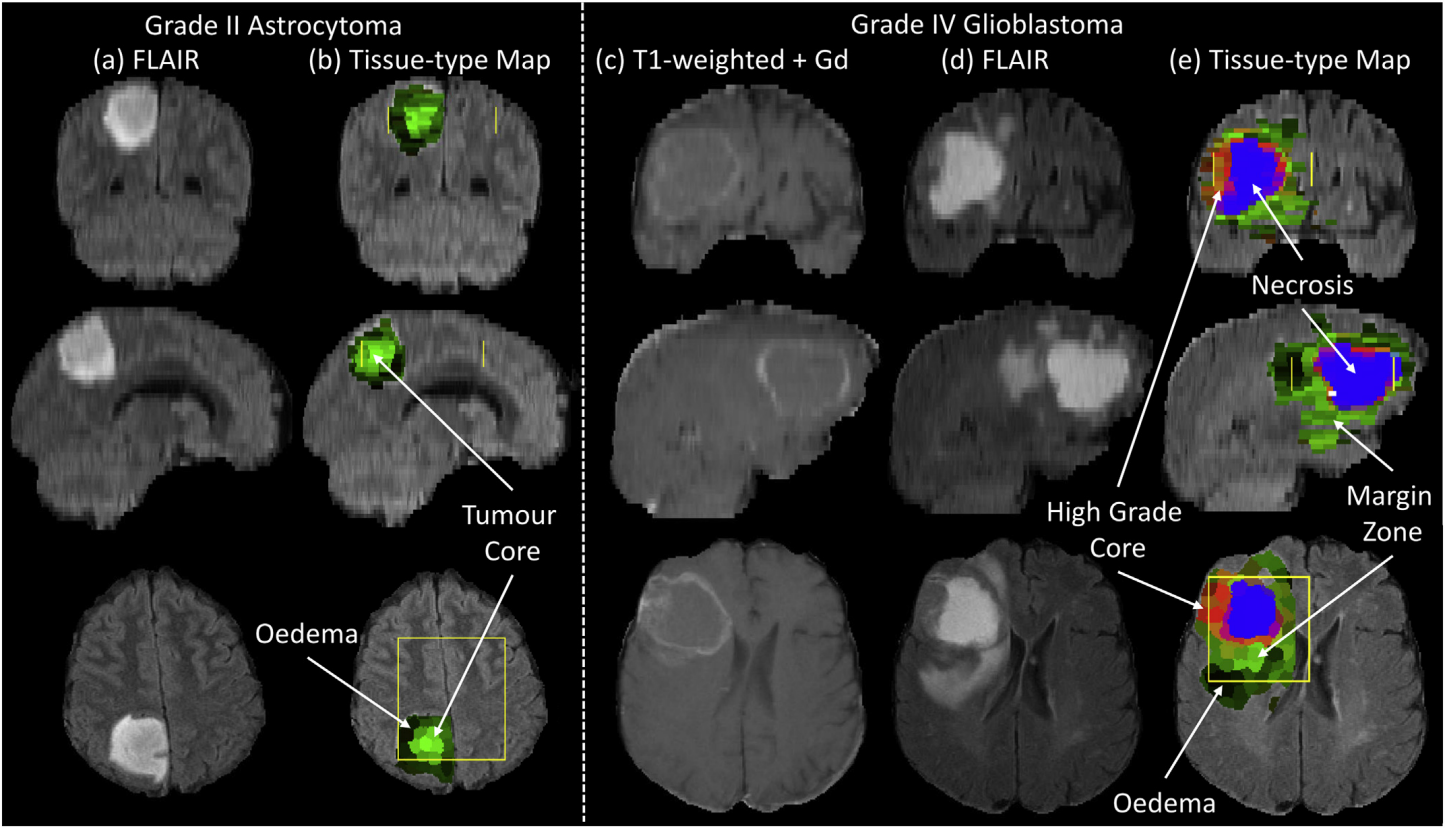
Whole brain 3D tissue-type maps of a grade II astrocytoma and grade IV glioblastoma. The astrocytoma example shows (a) FLAIR and (b) tissue-type colour maps in coronal, sagittal and axial slices through the tumour. The tissue-type colour map shows the extent of the tumour and identifies vasogenic oedema (black) surrounding a low-grade tissue tumour core (green). The margins of MRSI data acquisition are shown by the yellow lines on the tissue-type colour maps. The glioblastoma example shows (c) T1-weighted + Gadolinium contrast agent, (d) FLAIR and (e) tissue-type colour maps. The tissue-type map shows the extent of the tumour abnormality and includes the T1-weighted and FLAIR abnormality. With the proposed colour scheme, regions of tumour infiltration are represented as green, with regions exhibiting high-grade tumour features in red, necrosis in blue and vasogenic oedema as black. In (e) the whole of the green margin zone must be interpreted as tumour infiltration arising from a high-grade core.
